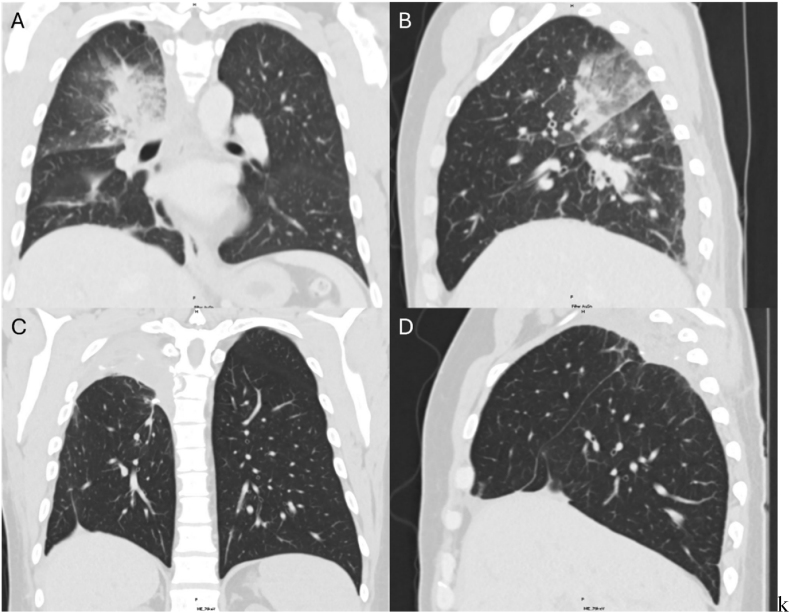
Right pulmonary mucormycosis before and after surgical lung resection. 2A is a coronal view of the patient's bilateral lung fields from his initial infectious workup, notable for consolidation in the perihilar posterior right upper lobe and superior segment of the right lower lobe with bronchial wall thickening and surrounding groundglass opacities. 2B depicts the right lung pathology from the same CT in a sagittal view. 2C and 2D are views from CT chest obtained approximately 2 months following right upper lobectomy and lower lobe segmentectomy showing a coronal view of the bilateral lung fields and a sagittal view of the right lung, respectively.
